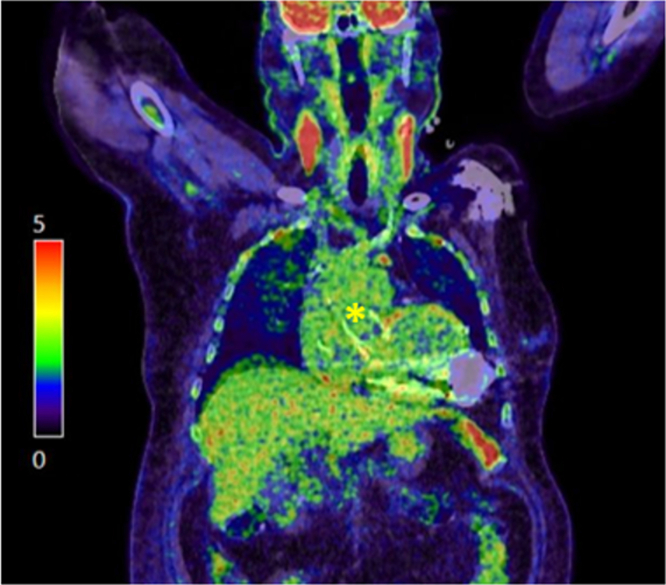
Case 2: PET/CT Fusion Image Showing Biodistribution of the Radiotracer (^18^F-Fluorodeoxyglucose) No focal pathological uptake was identified at the site of the transcatheter aortic valve prosthesis, particularly at the leaflet level (yellow asterisk), excluding endocarditis. PET/CT = positron emission tomography/computed tomography.
